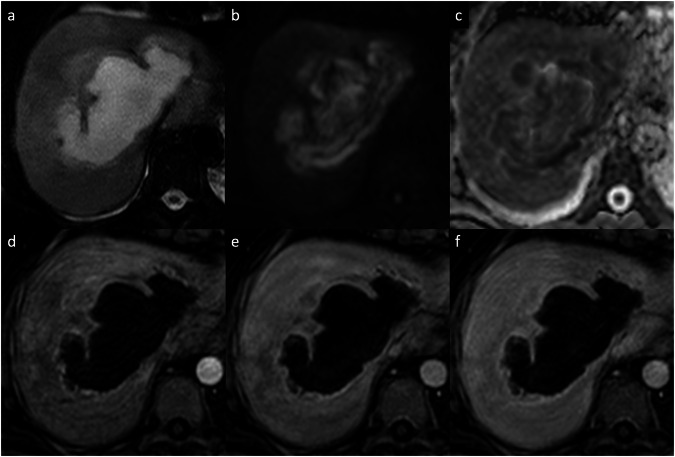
Amebic liver abscess in a 68-year-old man who presented with fever and right upper quadrant pain. Contrast-enhanced MRI using an extracellular contrast shows a large focal liver lesion, with (a) high SI on T2-weighted images, showing a “double target sign” with an iso- to hypointense inner layer and a hyperintense outer layer on T2-WI. The lesion shows (b) high SI on DWI (*b* = 800), (c) a peripheral low signal on the ADC map. d On the AP, the lesion demonstrates a thin-rim APHE, that persists (e) on the portal venous and (f) delayed phases
